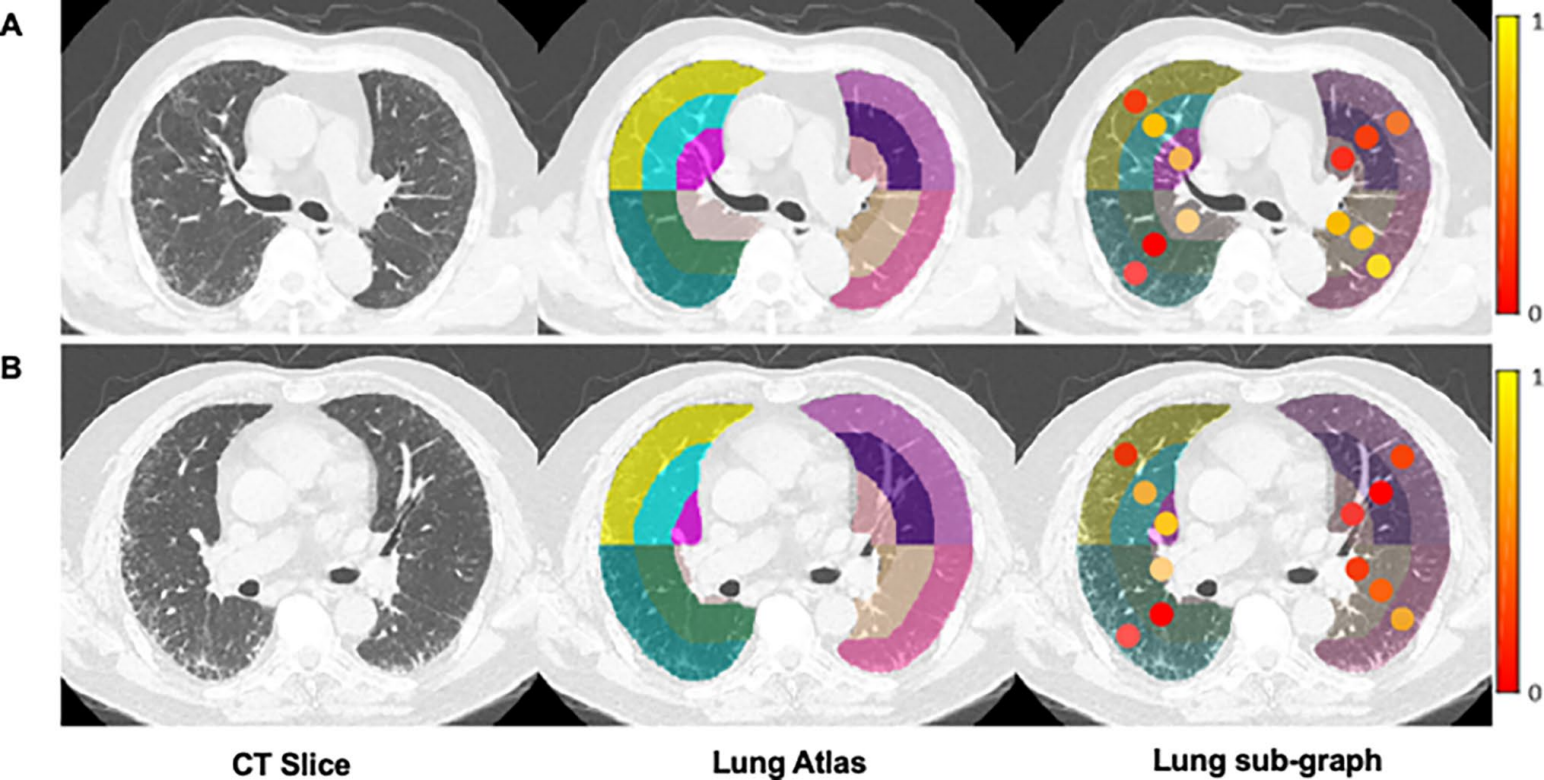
Two examples of lung graph construction. A A patient (male, age 78 years old) with non-fibrotic interstitial lung disease (NSIP). B A patient (male, age 70 years old) with fibrotic interstitial lung disease (idiopathic pulmonary fibrosis). From left to right: cropped CT slices of these two patients, the automatically generated lung atlas slices, the corresponding lung subgraph. This subgraph was obtained by the radiomics feature called log_sigma-1–0-mm-3D_glszm_SizeZoneNonUniformity. Each dots represents the strength of that feature in the subgraph. For better visualization, the values of each sub-region were scaled to (0,1)
